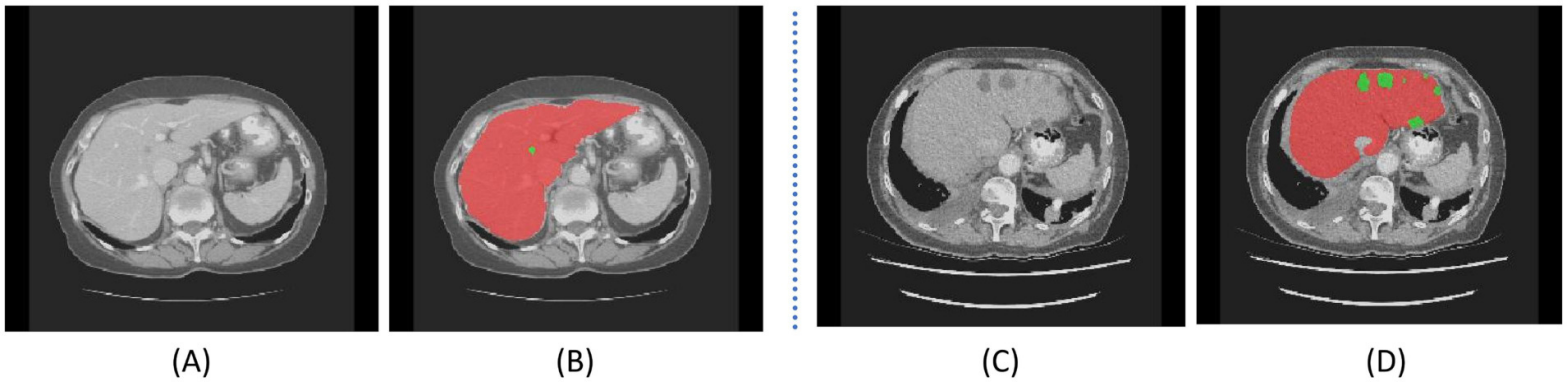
CT scan shows significant changes in the shape, size, and location of liver lesions. (A) and (C) display CT scan results obtained from individual patients, while (B) and (D) are labeled results, where green area represents tumor and red represents liver.
